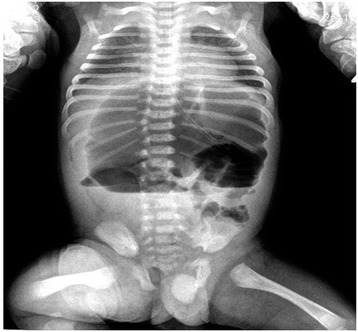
Abdominal X-ray showing a distended large bowel loop with air-fluid levels.
